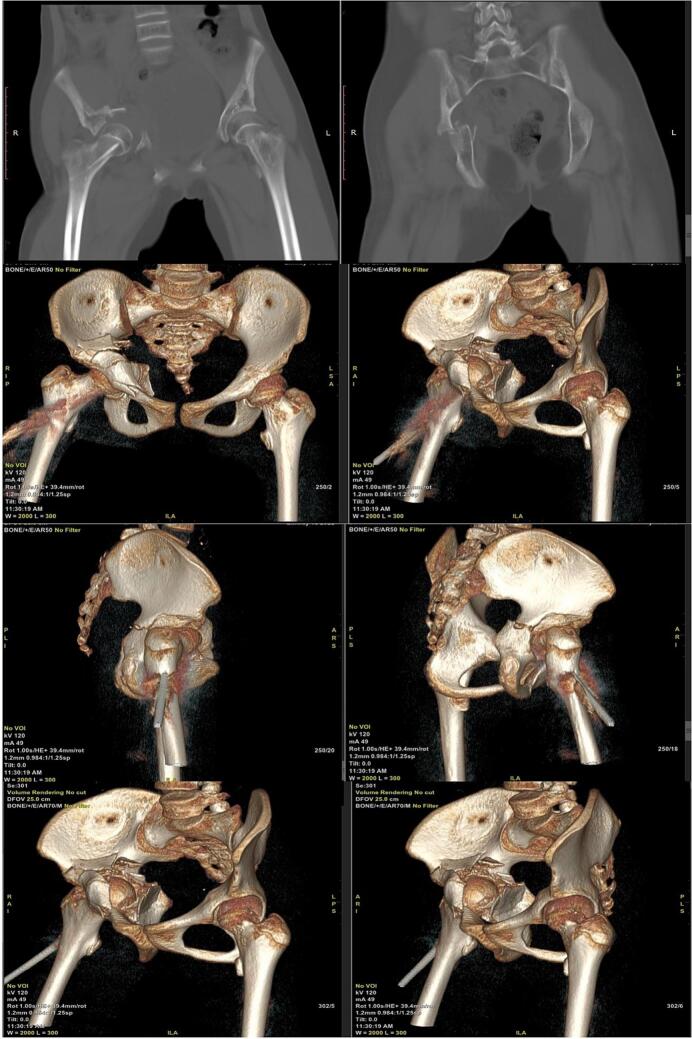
The reconstruction 3D-CT scan of the pelvis showing a severe degree of both column acetabular fracture with central hip dislocation.
